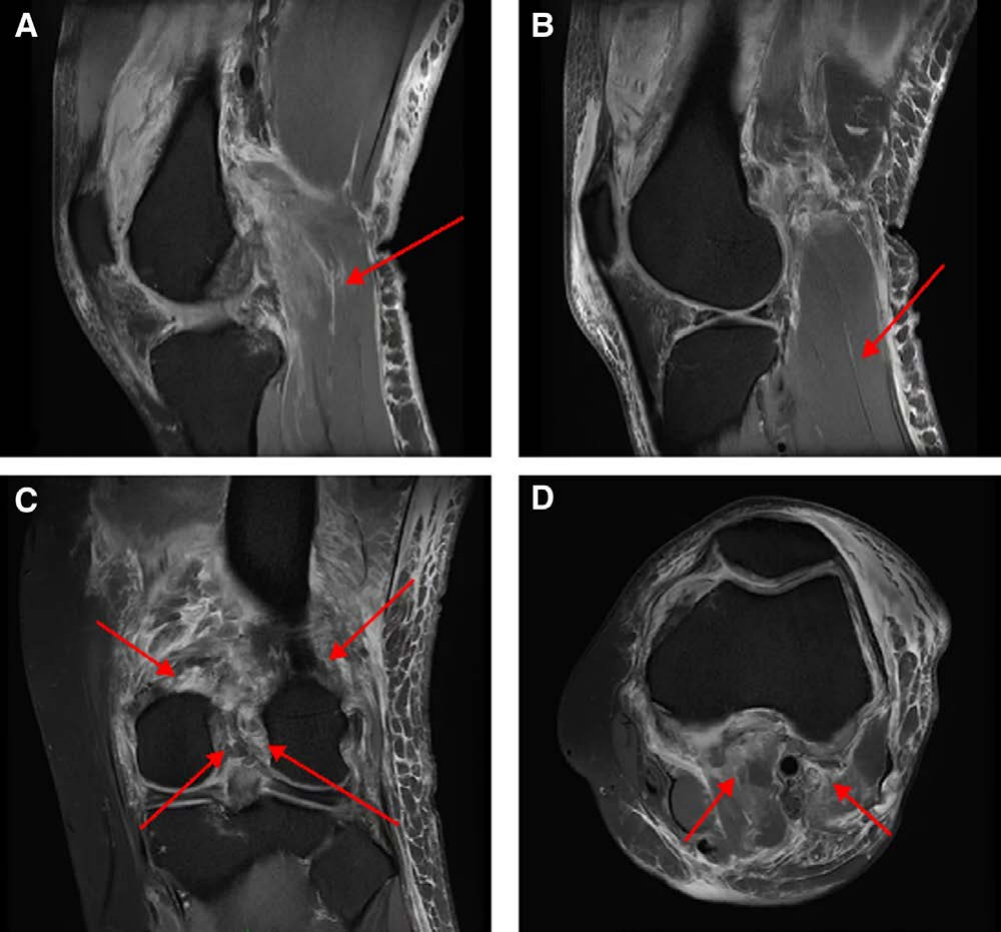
Proton density magnetic resonance images 2 days after the car accident, (A) sagittal image of the ruptured medial head of the gastrocnemius muscle (arrow), (B) sagittal image of the torn lateral head of the gastrocnemius muscle (arrow), (C) coronal images of the ruptured medial head, lateral head, posterior cruciate ligament and anterior cruciate ligament of the gastrocnemius muscle (arrow, from top to bottom, from left to right), (D) axial images of the ruptured medial head and lateral head of the gastrocnemius muscle (arrow, from left to right).
